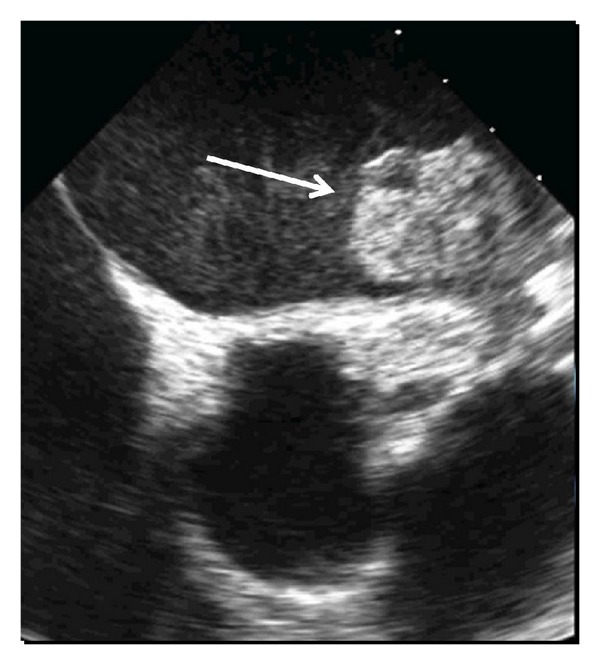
Transesophageal echocardiographic image of a large thrombus (arrow) on a WATCHMAN device several months after anticoagulation was discontinued.
